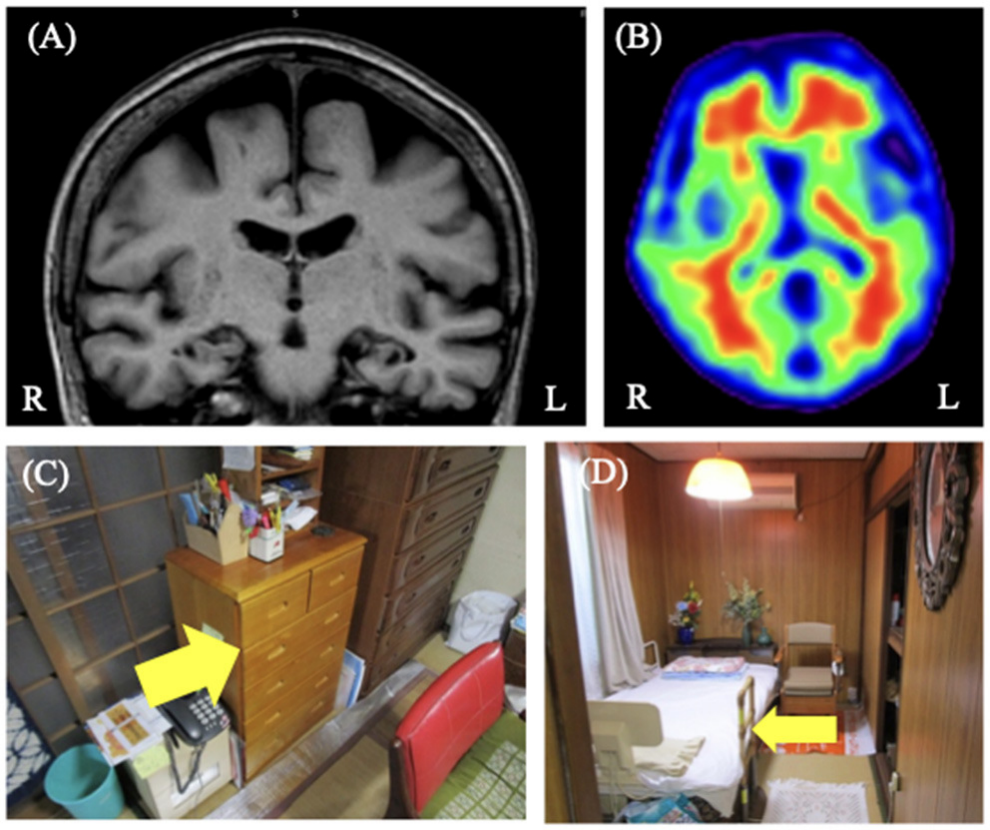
Case 1 images. (A) Brain magnetic resonance T1-weighted image showed diffuse cortical atrophy but relative sparing of the medial temporal lobes with bilateral hippocampal sulcus remnant. (B) Florbetapir 18 positron emission tomography showing positive results. (C,D) These are the images of the patient's home. She usually stored her valuables such as wallet in yellow arrowed shelf (C). She sometimes hid her valuables under the yellow arrowed bed (D). We proposed to her family that they put her valuables back to usually stored place if they found them in unusual places.
